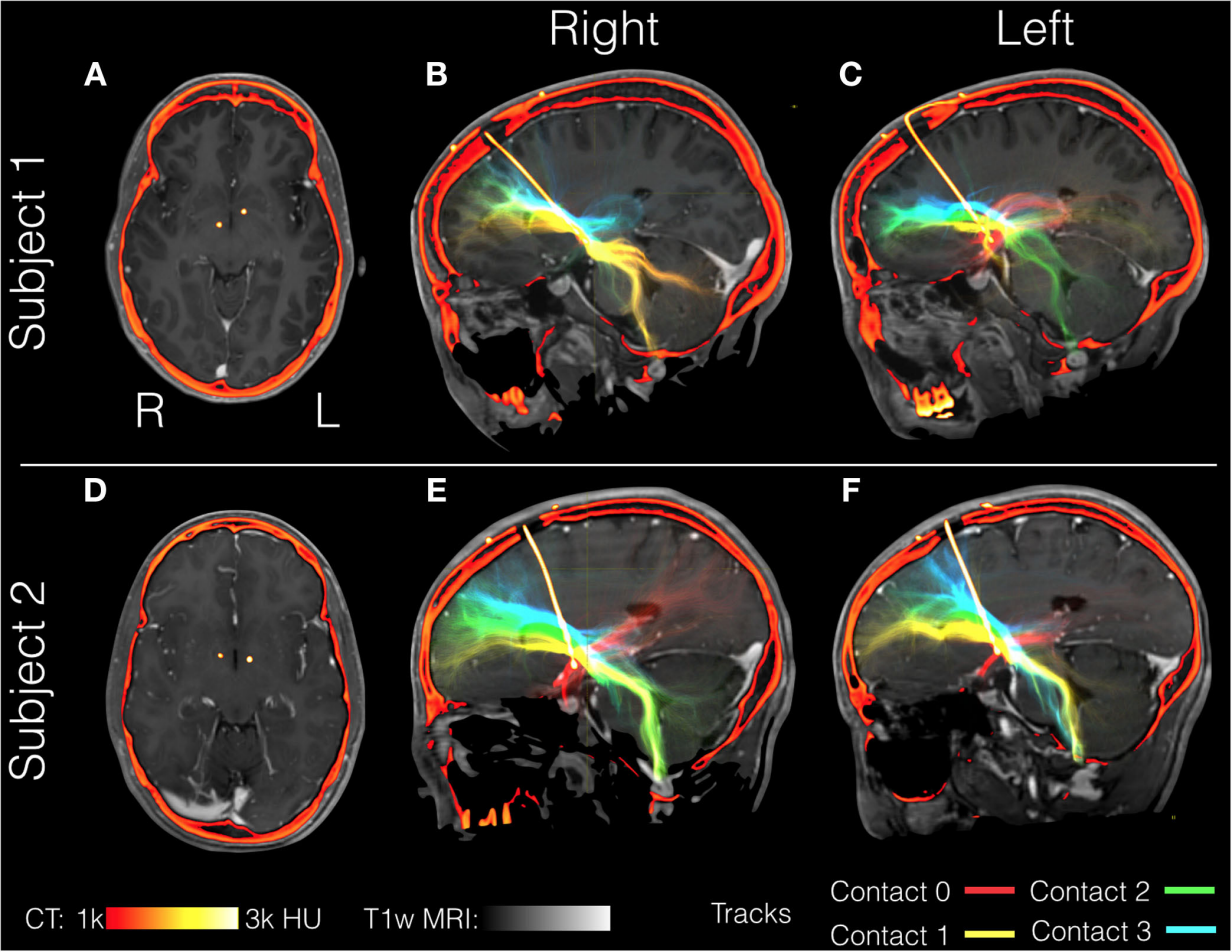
Individual patient tractography based on electrode location in OCD. Tractography from contacts in two patients with obsessive compulsive disorder (OCD), Subject 1 (A–C) and Subject 2 (D–F). Fiber tracts are color-coded starting from the most distal: contact 0 (red), contact 1 (yellow), contact 2 (green), and contact 4 (blue). Structural T1-weighted MRI is shown in gray, with postoperative computerized tomography (CT) shown in hot colors depicting the skull and the electrode. Axial views show the location of the most distal electrodes (A,C) while the sagittal views show the entire electrode including the point it enters the burr hole at the top of the image (B,C,E,F).
